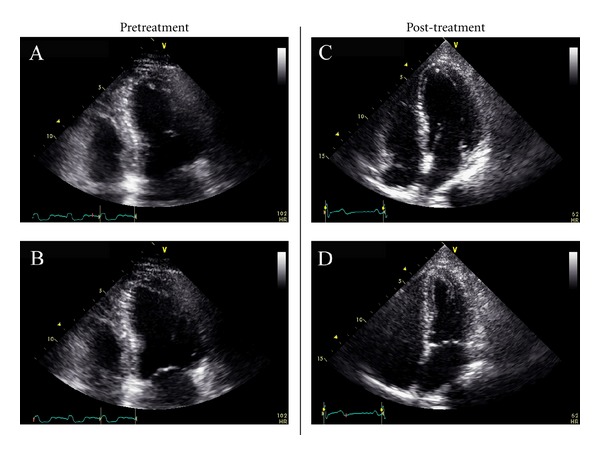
Transthoracic echocardiogram before and after treatment with IVIG and steroids. Apical 4-chamber view with pretreatment end diastole (A) and end systole (B) demonstrating severe global systolic dysfunction, LVEF <20%. The repeat transthoracic echocardiogram before treatment prior to discharge end diastole (C) and end systole (D) demonstrating a near complete resolution of ventricular systolic dysfunction.
